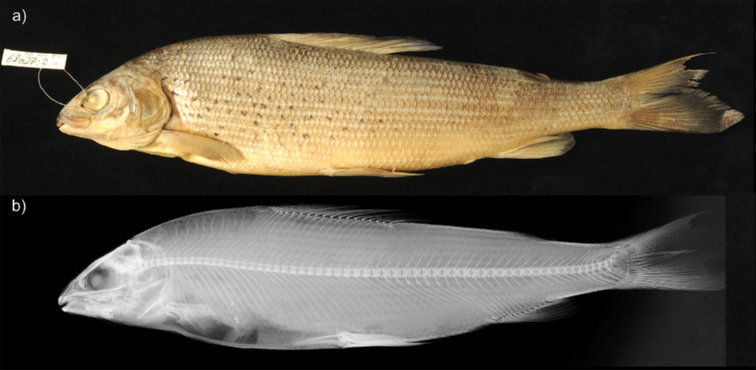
Photo (a) and radiograph (b) of the neotype *Thymallus
